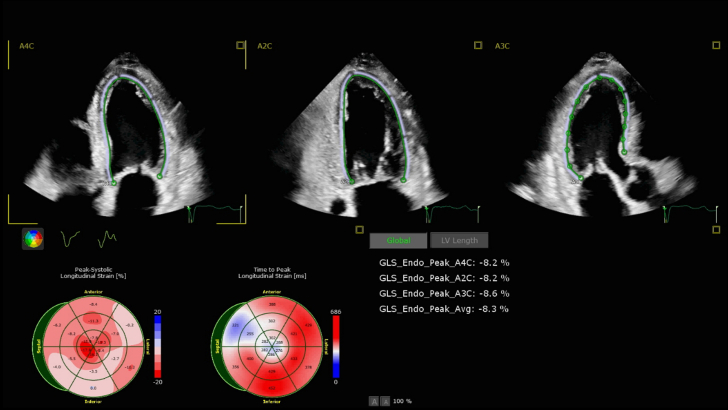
Transthoracic Echocardiography Strain From First Hospitalization Global longitudinal strain is reduced.
